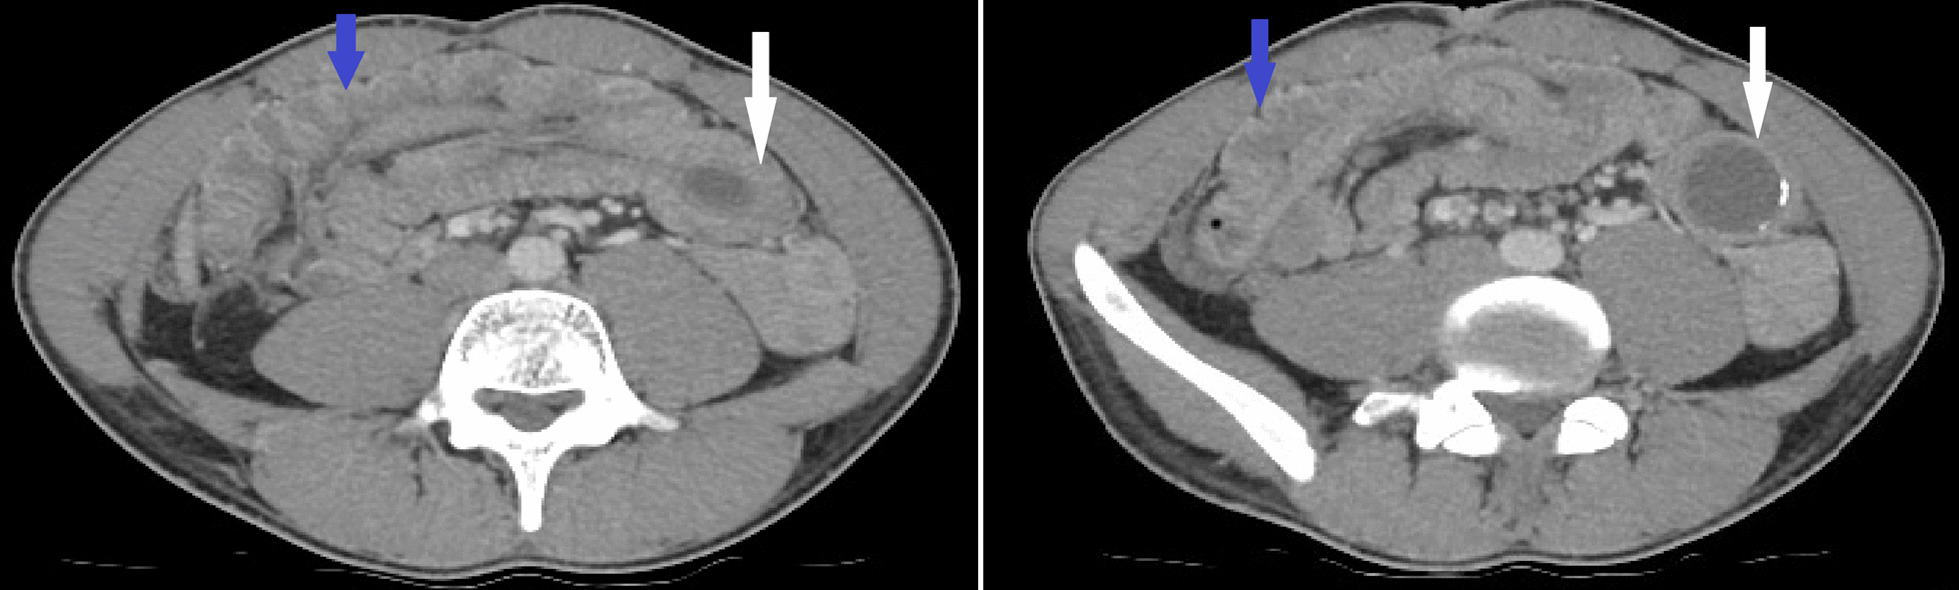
Axial CT scan demonstrated the ileocecocolic intussusception (blue arrows) and large appendiceal mucocele (white arrows)
